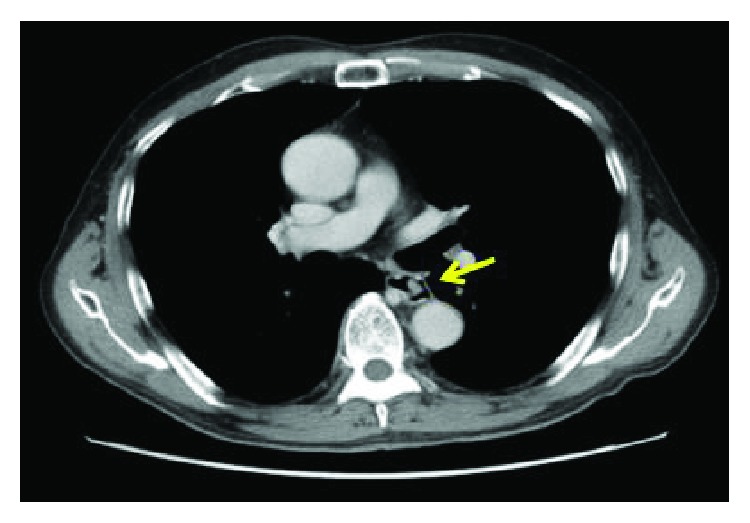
Chest computed tomography showing an elevated mass in the esophageal lumen (arrow).
